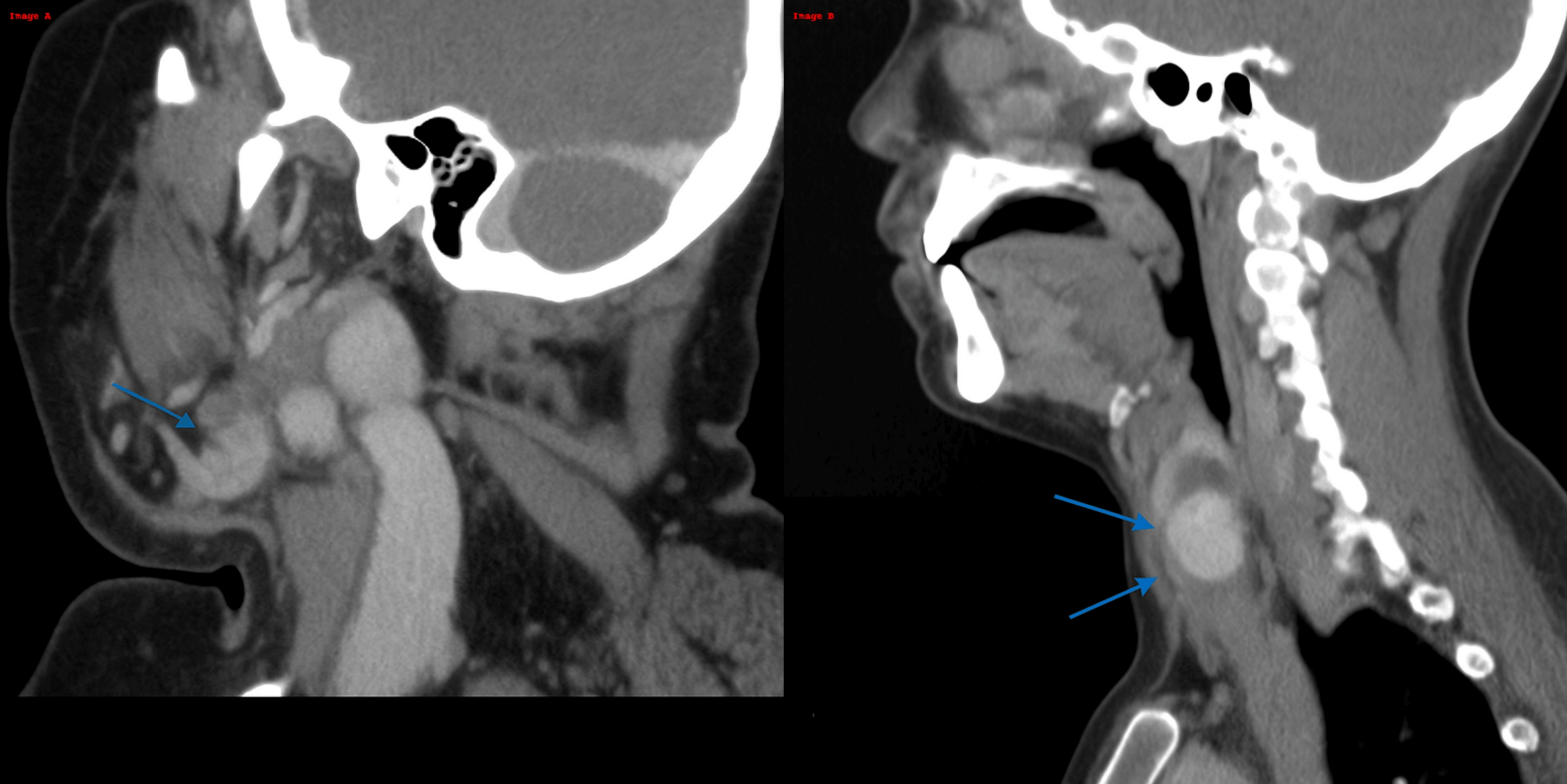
Contrast-enhanced sagittal CT showing a hypodense, rim-enhancing collection consistent with a retropharyngeal abscess. Image A: Contrast-enhanced sagittal CT image showing a well-defined, hypodense, rim-enhancing collection (blue arrows) in the anterior neck region, consistent with a retropharyngeal abscess. Image B: Higher sagittal CT slice demonstrating a similar hypodense lesion near the skull base and parapharyngeal space (blue arrow), raising concern for contiguous spread.
